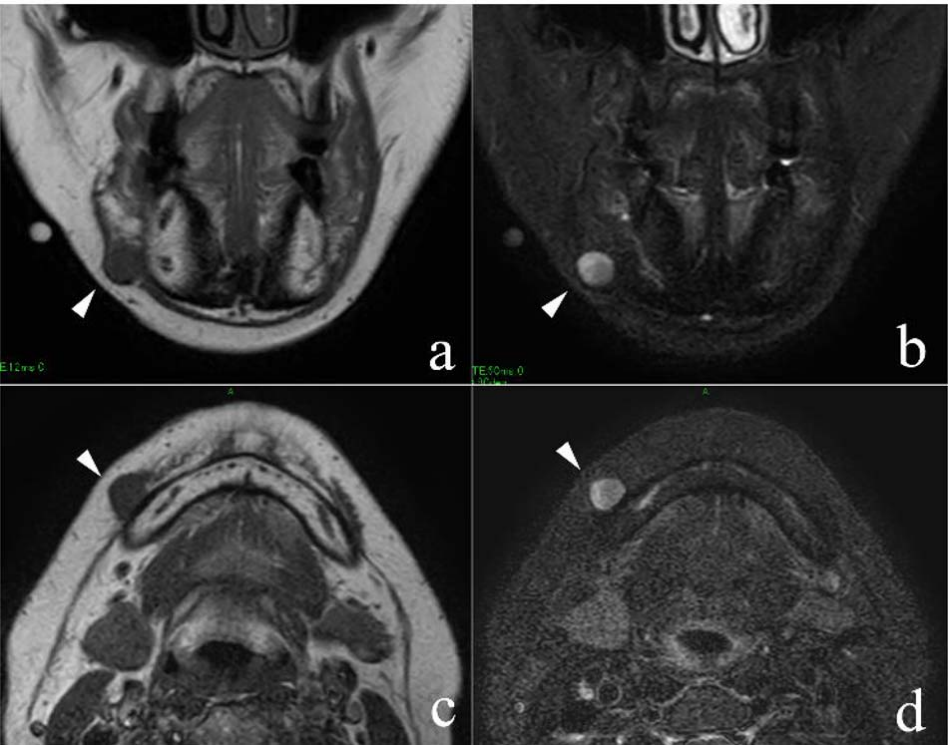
MRI findings; the arrowheads indicate the schwannoma; (a) low signal intensity on T1-weighted coronal image; (b) high signal intensity on T2-weighted coronal image; (c) low signal intensity on T1-weighted axial image; (d) high signal intensity on T2-weighted axial image.
